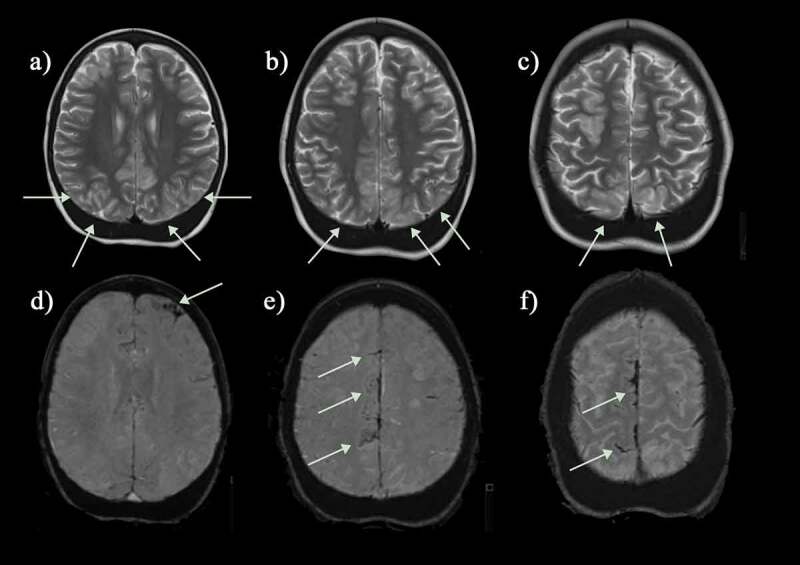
This teenager presented with sudden onset of blindness and seizures soon after acute chest syndrome. (a-c) There is occipital and frontal cortical and subcortical swelling on T2-weighted MRI, consistent with posterior reversible encephalopathy syndrome (green arrows) and (d-f) the susceptibility weighted imaging (SWI) revealed hemorrhage in the parafalcine and left frontal regions (green arrows). The magnetic resonance angiograms was normal. The patient returned to mainstream school but developed epilepsy
